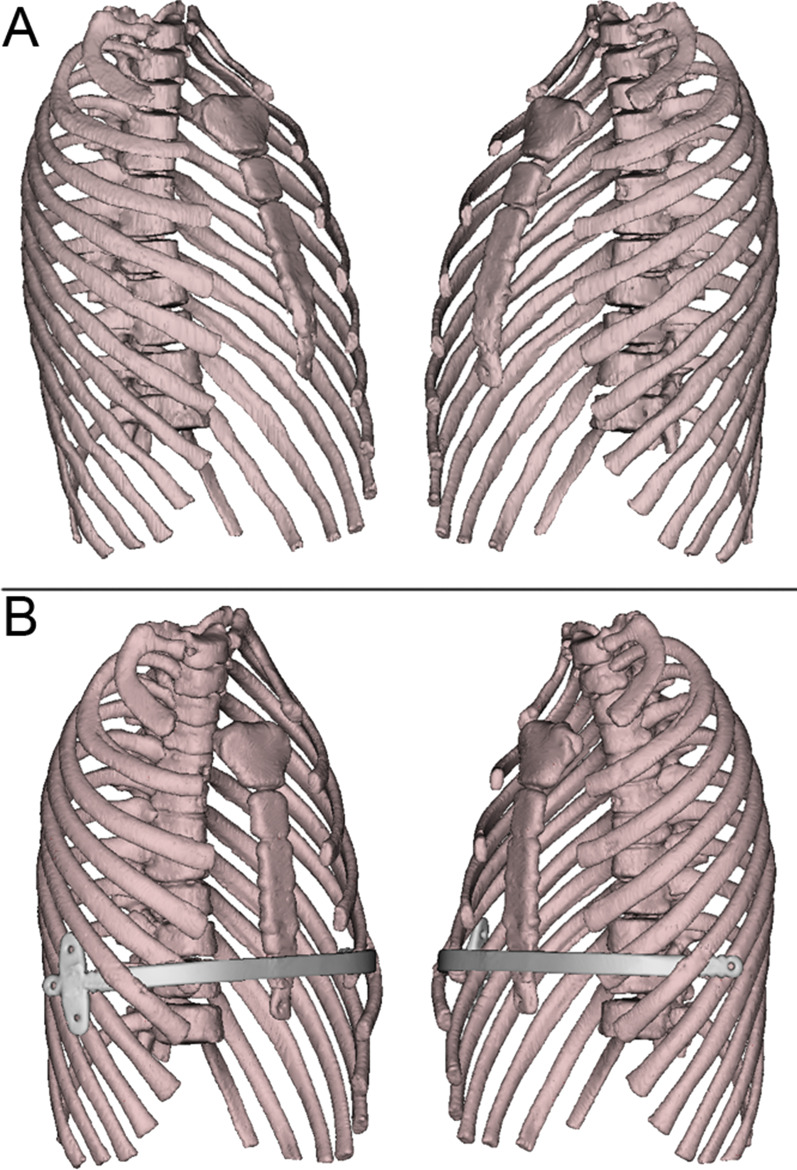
The 3-dimensional imaging of a 13-year-old patient’s computed tomography before a and after b the operation
